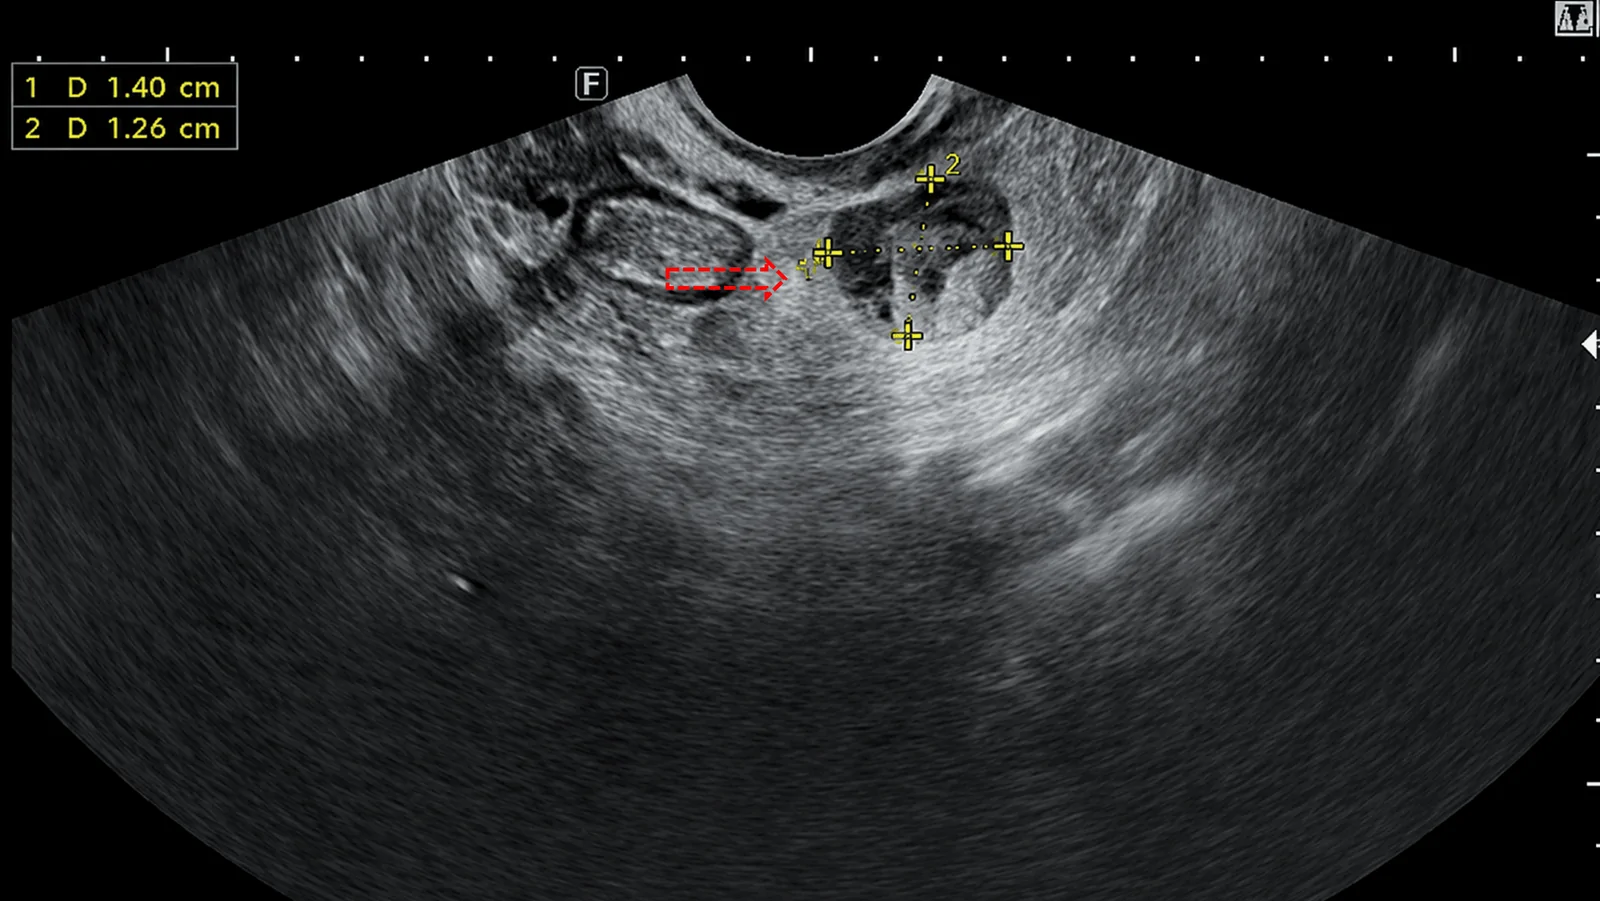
Case 1: transvaginal ultrasound Transvaginal ultrasonography of Case 1, demonstrating a gestational sac-like structure (red arrow), measuring 1.40 × 1.26 cm, in the left pelvic cavity remote from the left fallopian tube.
